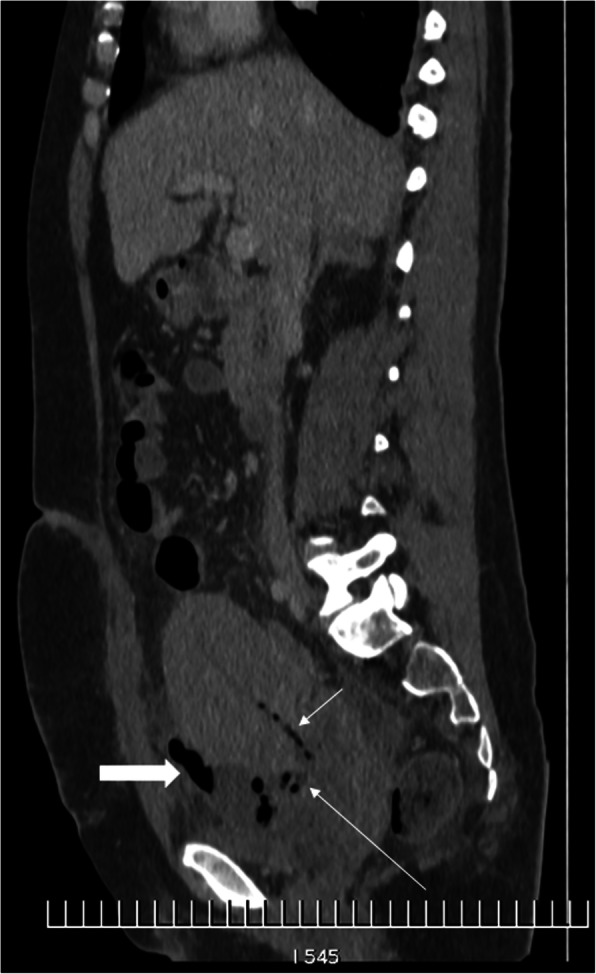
A 33-year-old woman 6 days after repeat C-section in 2nd pregnancy. CT is performed due to fever, pain, left costovertebral angle tenderness, and increasing serum level of C-reactive protein (485 mg/l) and procalcitonin (6.7 ng/ml), with a suspicion of renal colic or endometritis. CT revealed gas within the C-section incision in the uterine wall (long arrow) and in the uterine cavity (short arrow), as well as an abscess in front of/under the uterus (thick arrow), shifting the bladder to the left. Two hours later, the patient was operated on, and the abscess was evacuated
